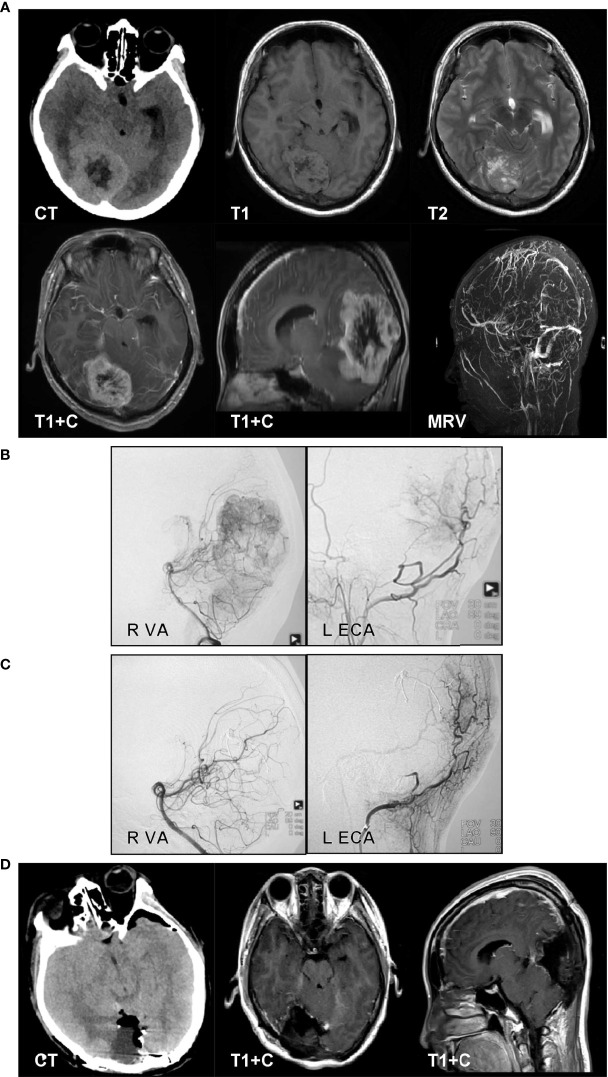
A 33-year-old man complained of headache for 2 months. The CT results revealed a mixed-density, right occipital lesion. The MRI results revealed a right occipital, mixed-signal lesion and flow voids on T1- and T2-weighted imaging. The lesion showed obvious enhancement on contrast-enhanced MRI. The magnetic resonance venography results showed an obstructed sagittal sinus (A). We performed the operation in a hybrid operating room. The cerebral digital subtraction angiography (DSA) results revealed abundant tumor blood. The feeding arteries were the right posterior cerebral artery (PCA), right external carotid artery (ECA), right meningiohypophyseal trunk, and left ECA (B). Onyx-18 was used for feeding artery embolization for the right PCA, right occipital artery, and left occipital artery. The DSA reexamination results showed that 90% of the blood supply was embolized (C). Craniotomy was then performed. The occipital interhemispheric transtentorial approach was used to reach the tumor under navigation. The tumor was tenacious, with partial calcification. Piecemeal removal was performed, and we achieved a Simpson grade II resection. The postoperative CT results showed no hemorrhage or infarction (D). The pathology examination results revealed that the tumor was a hemangiopericytoma.
